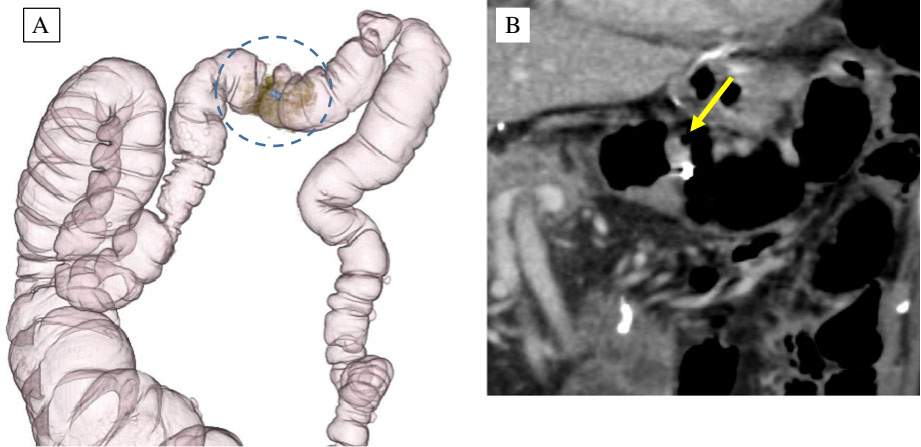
a, b Images from abdominal enhanced computed tomography (CT). CT revealed an increased wall thickness along the left half of the transverse colon [indicated by a *dotted line* (a) and *arrow* (b). However, the lack of increased CT values in the surrounding fatty area suggests that the invasion depth was the muscularis propria (MP) layer. No lymph node or distant metastasis was observed
